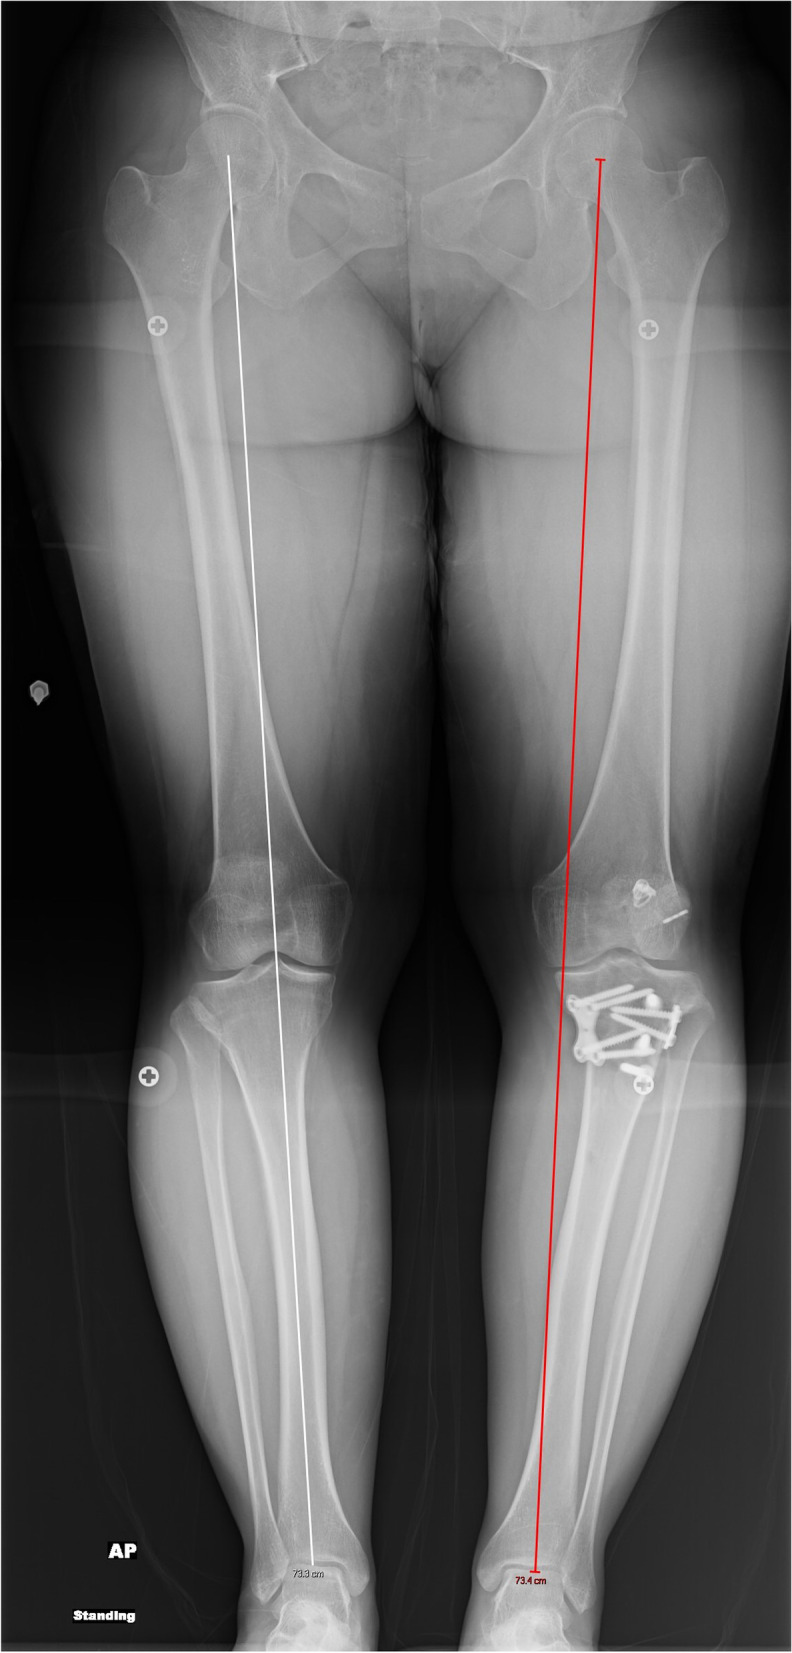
A post-operative standing mechanical axis radiograph demonstrating varus malunion of the anterior closing wedge osteotomy
